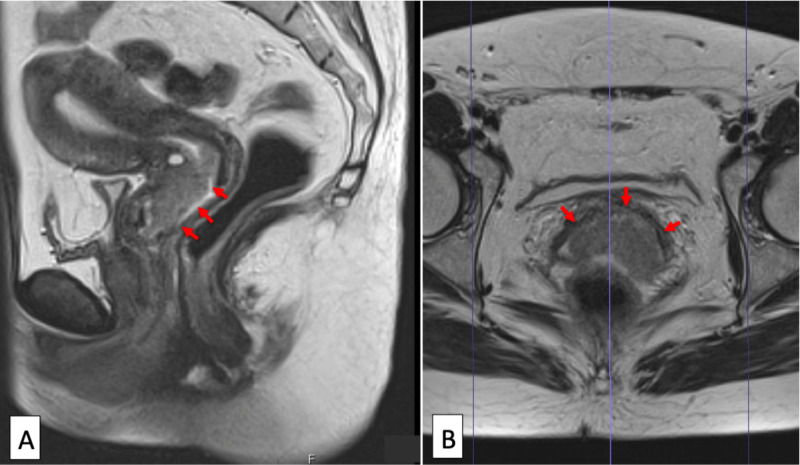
Initial magnetic resonance imaging of uterine cervix tumor Sagittal (A) and axial (B) magnetic resonance images showed an irregular-shaped uterine cervix tumor (arrows).
